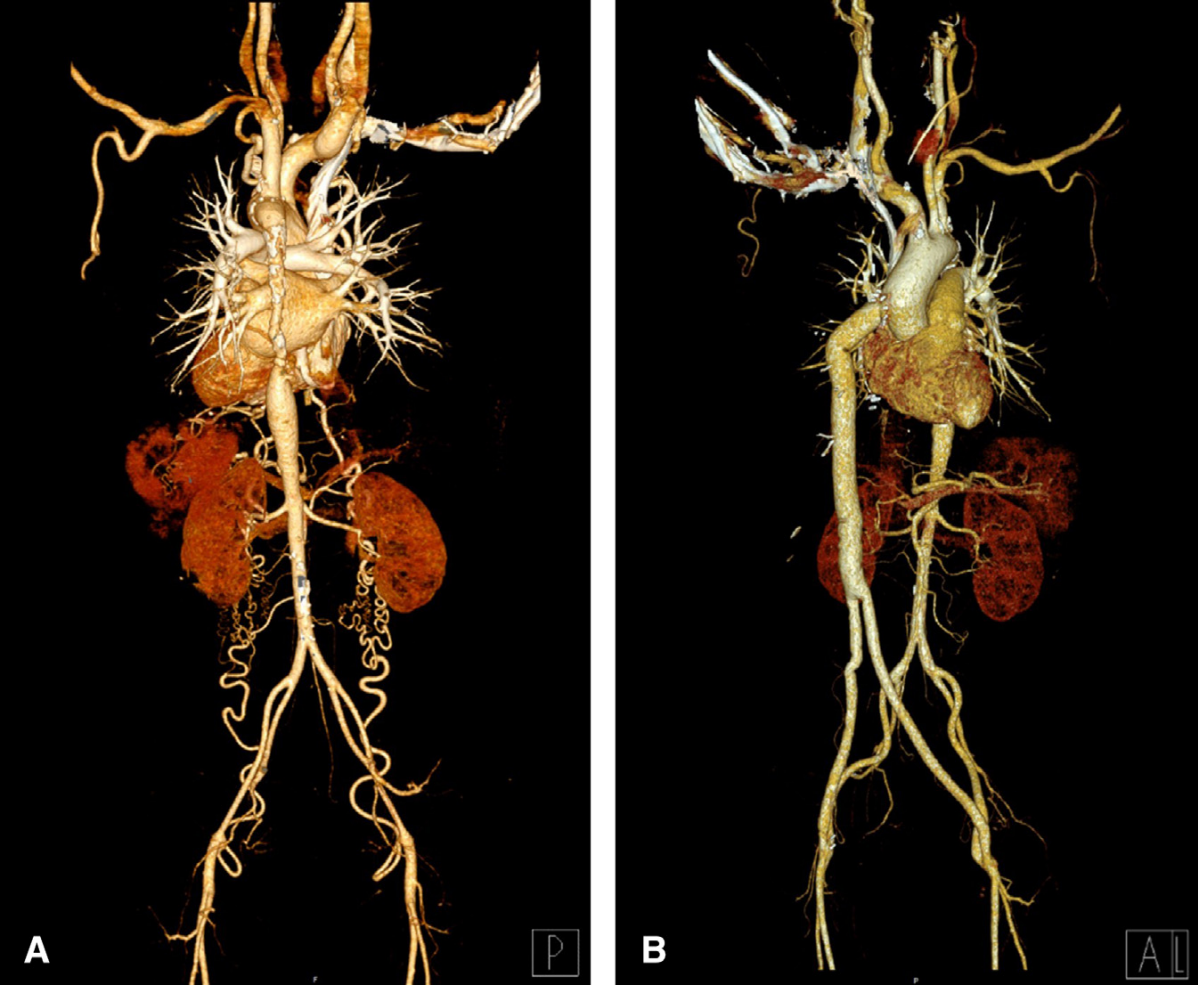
Three-dimensional computed tomography images of patient No. 2. A, Preoperative computed tomography reveals a diffuse narrowing of descending thoracic aorta (minimal diameter: 6.5mm) with multifocal wall calcification observed in the posterior view. B, Postoperative computed tomography reveals a patent bypass graft in the anterior view.
